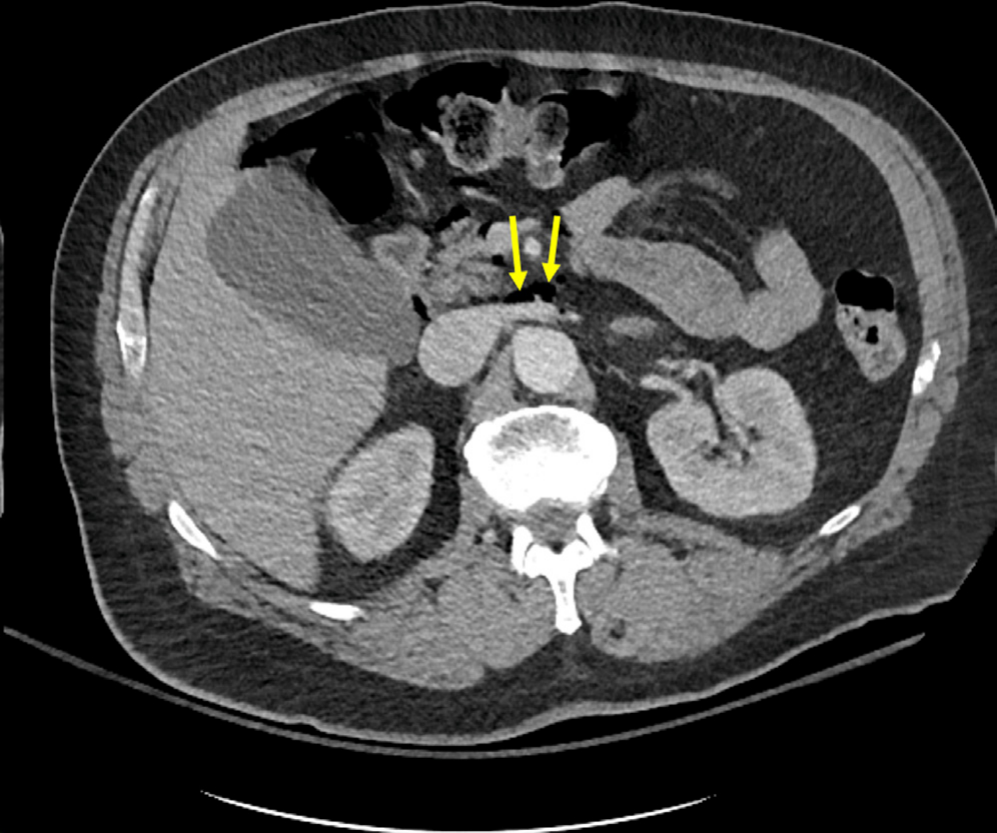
Computed tomography of the abdomen with intravenous contrast demonstrating a small volume of retroperitoneal air (*yellow arrows*).
